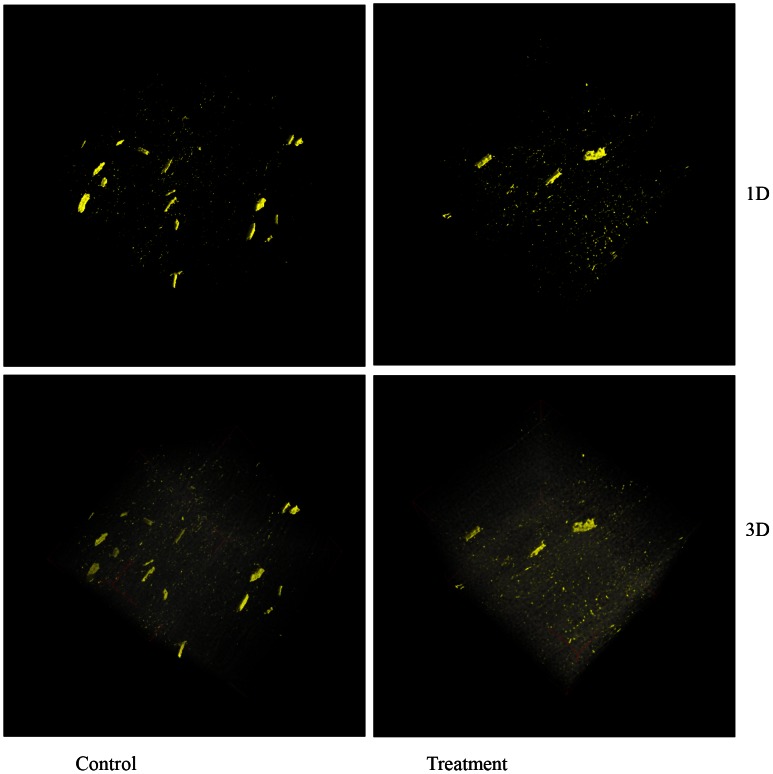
X-ray computed tomography analysis. Two-day-old soybean plants were flooded for 2 days, after which the cotyledon was collected and fixed. Untreated plants were used as control. Digital geometry processing was used to generate a three-dimensional image of the inside of the cotyledon from a large series of two-dimensional X-ray images taken around a single axis of rotation. The “1D” and “3D” show the first-dimension and three-dimension.
